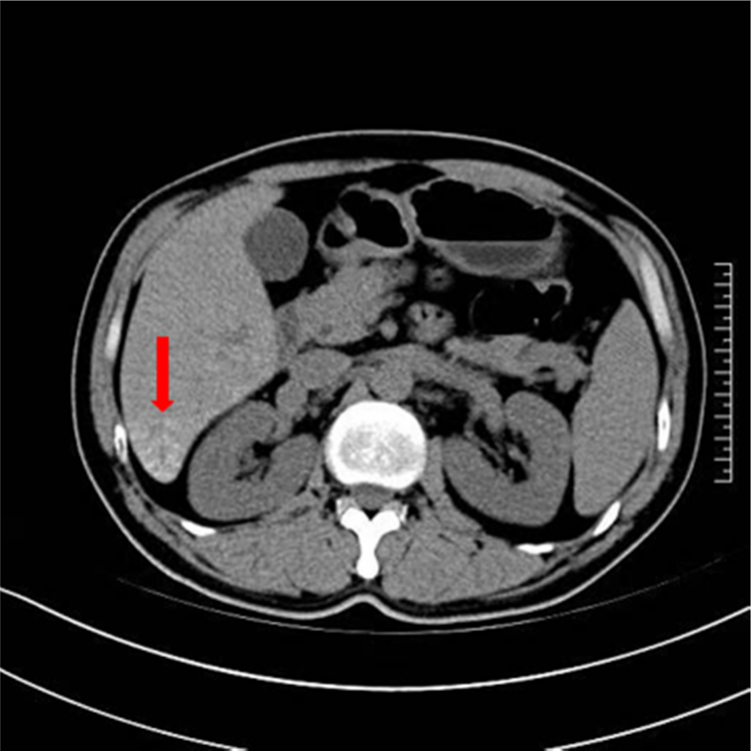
CT-scan showing a little iodized oil deposition shadows.
